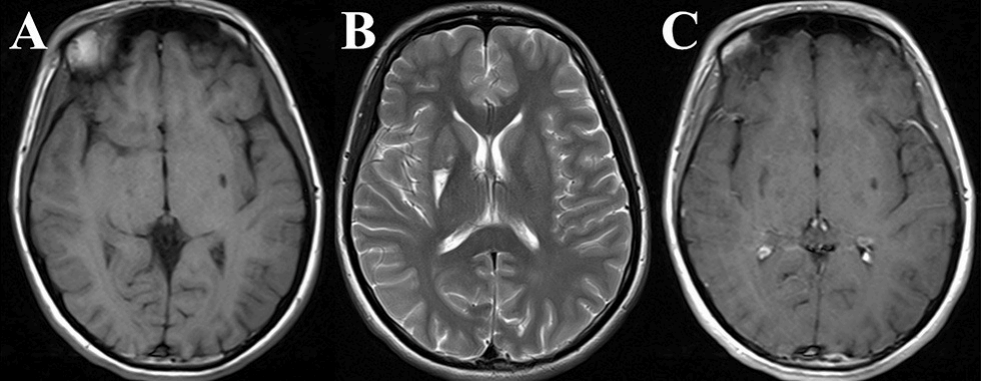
The proband's brain magnetic resonance imaging (MRI) obtained at 18 years old. Axial T1/T2-weighted images [Panels (A) and (B), respectively] and contrast-enhancement T1-weighted image (C) of the brain were obtained when the proband appeared with the first seizures. An apparent large lesion was seen in the right putamen, and a relatively small lesion was seen in the left putamen.
